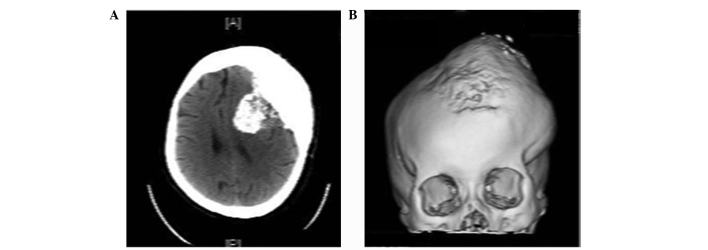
(A) Head CT virtual endoscopy scan showed a large density in the bilateral frontoparietal bone, particularly in the left side, with an unclear boundary and uneven density, measuring ~140×120 mm. (B) Head CT showed a cone-shaped intracranial meningioma below the large left frontoparietal bone density, measuring ~80×60×30 mm, which had evidently shifted the midline structure to the right. CT, computed tomography.
